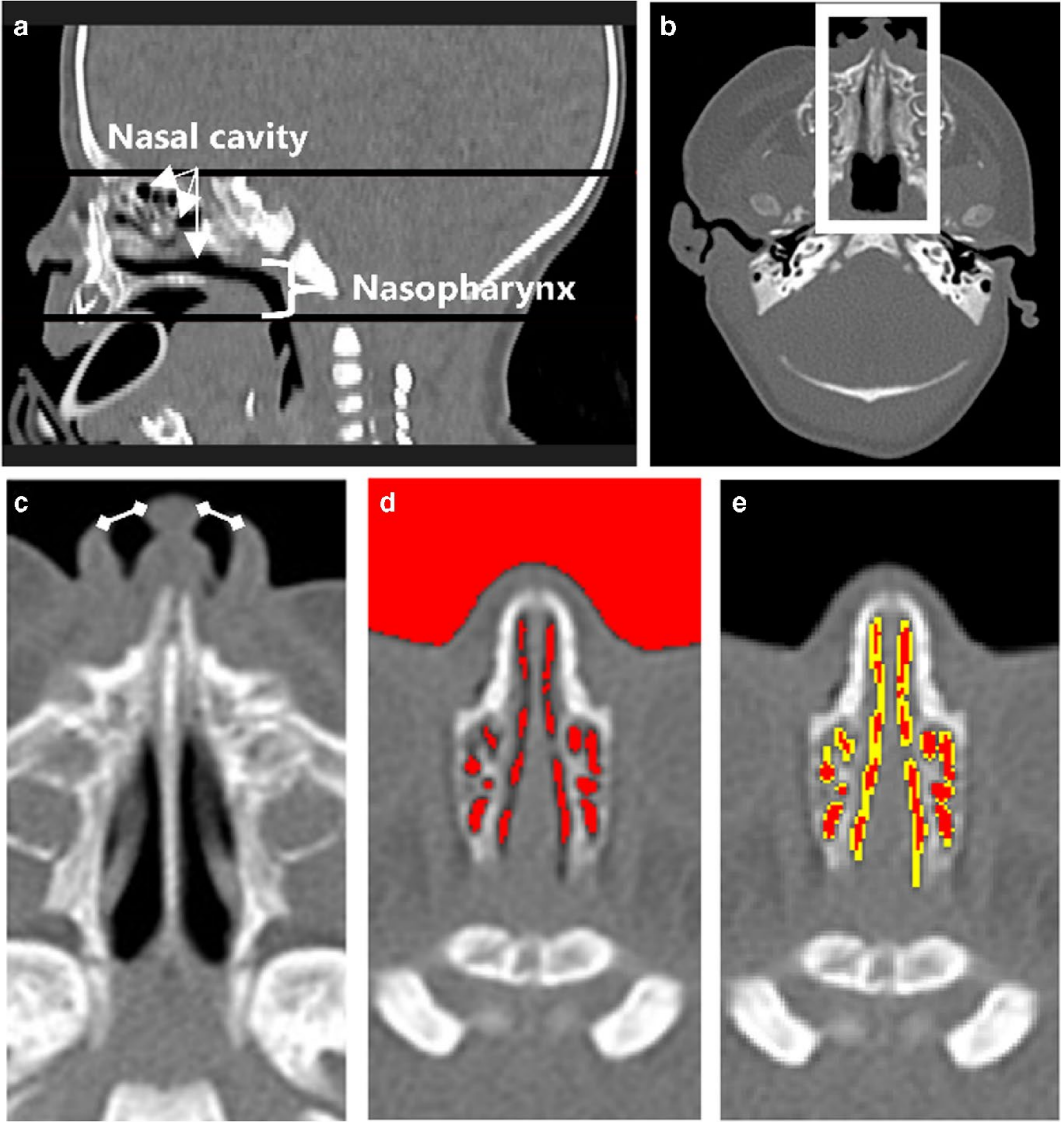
Automatic nasal airway segmentation process in an 8-day-old boy (computed tomography (CT) scan without contrast). a, b Selection of the volume of interest (VOI). a Reconstructed sagittal view showing the axial slices (*black lines*) that define the upper and lower boundaries of the nasal cavity and the nasopharynx, automatically selected by the algorithm. b Axial slice showing automatic cropping of the nasal airway region; a *white box* indicates the region of interest. c–e Nasal airway segmentation in axial view. c Nostril borders are defined by *straight lines* (*white lines*). d Initial segmentation using a global threshold of − 400 Hounsfield units (*red*, algorithm output) includes surrounding air, while narrow regions remain undetected. e Final segmentation after applying local adaptive thresholding and 3-connectivity filtering to include narrow regions (*yellow* indicates added areas, *red* indicates the initial segmentation; both are outputs of the automatic algorithm). The surrounding air was automatically removed
